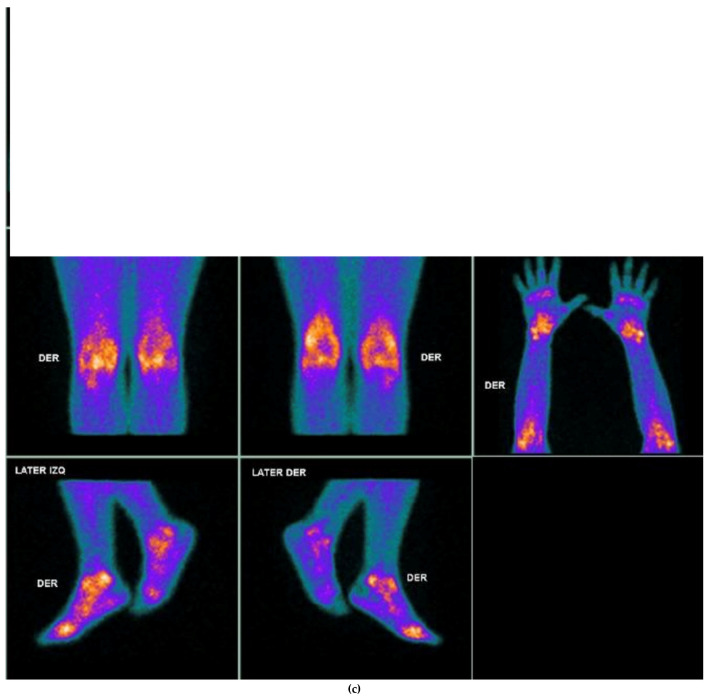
^99m^Tc HYNIC-TOC scintigraphy showing abnormal uptake of the radiotracer surrounding the breast implants (a) and knees, elbows, carpal joints, and ankles (c). Postexplantation scintigraphy showing no uptake in surgical bed in the same patient (b).
